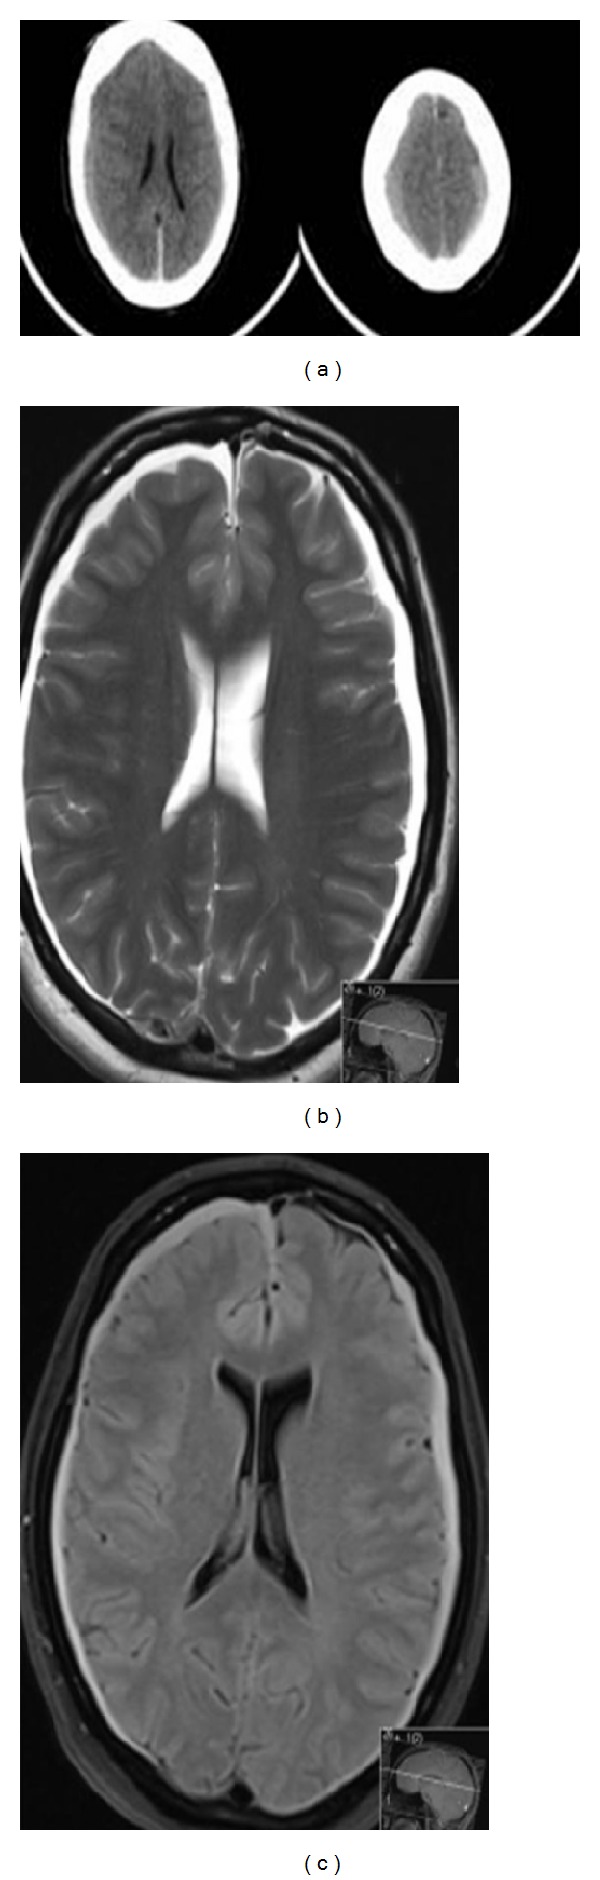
(a) Noncontrast head CT: bilateral hematomas are present. These are predominantly isodense to slightly hypodense compared to the adjacent gray matter (29–35 Hounsfield units), which could be explained by anemia (hemoglobin was 9 g/dL). (b) and (c) Bilateral subdural hematomas, confirmed as shown in both MRI T2 and FLAIR sequences.
